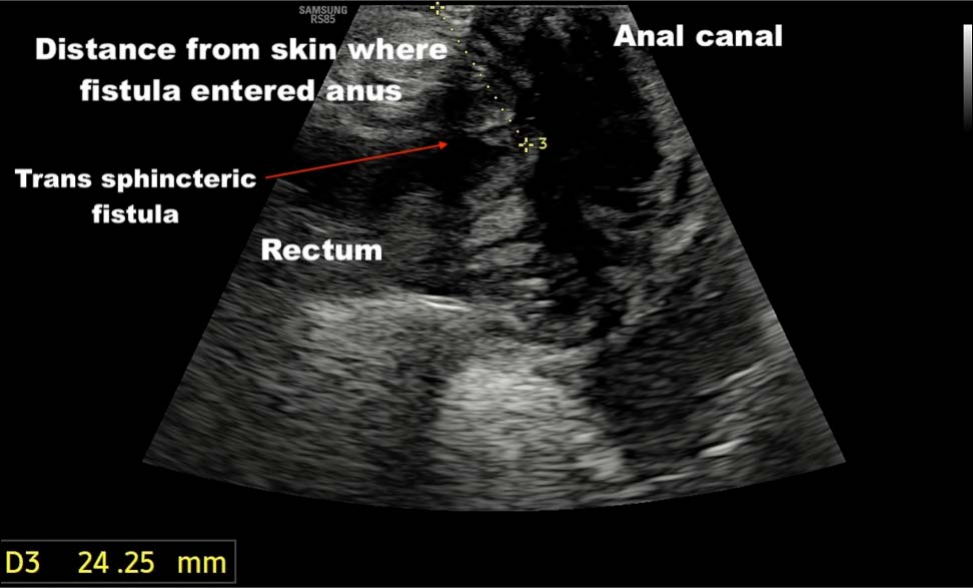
Transperineal ultrasound showing trans-sphincteric fistula tract originating from the 6 o'clock position of the anal canal.
